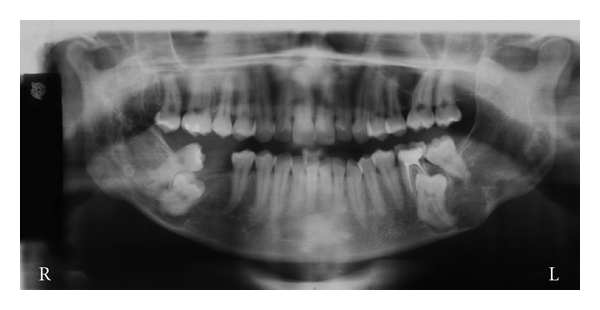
Panoramic radiograph revealing formation and replacement of the lesion by neoformed bone tissue, and the right mandibular ramus was larger than the left side.
